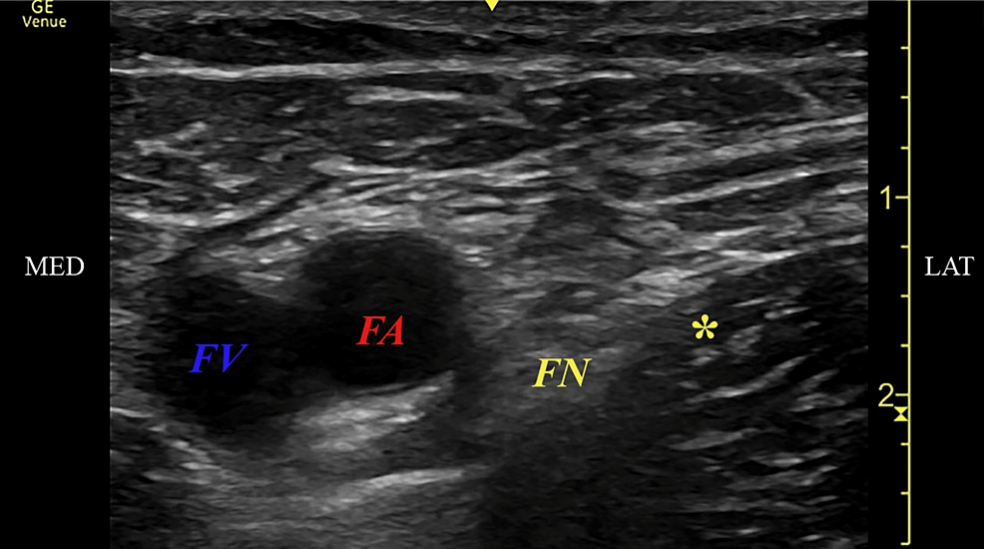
Ultrasound image of the femoral triangle using a linear array transducer Ultrasound of the femoral nerve (FN) at the inguinal crease. * Denotes the final location of the peripheral nerve stimulator (PNS). FA= femoral artery, FV= femoral vein, MED= medial, LAT= lateral
